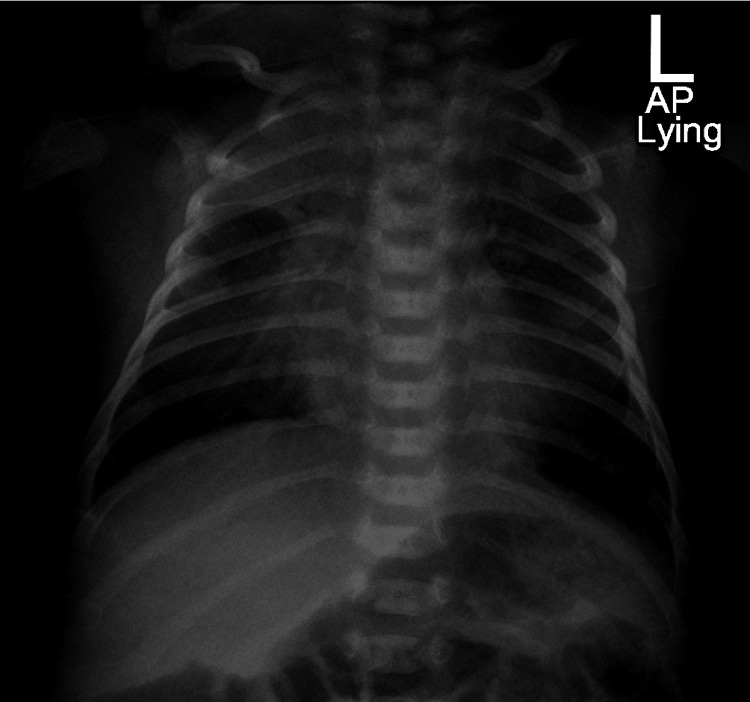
Chest X-ray (anterioposterior view) on admission showing right upper lung zone consolidation, diffuse interstitial infiltrates, and accentuated bronchovascular markings bilaterally.
